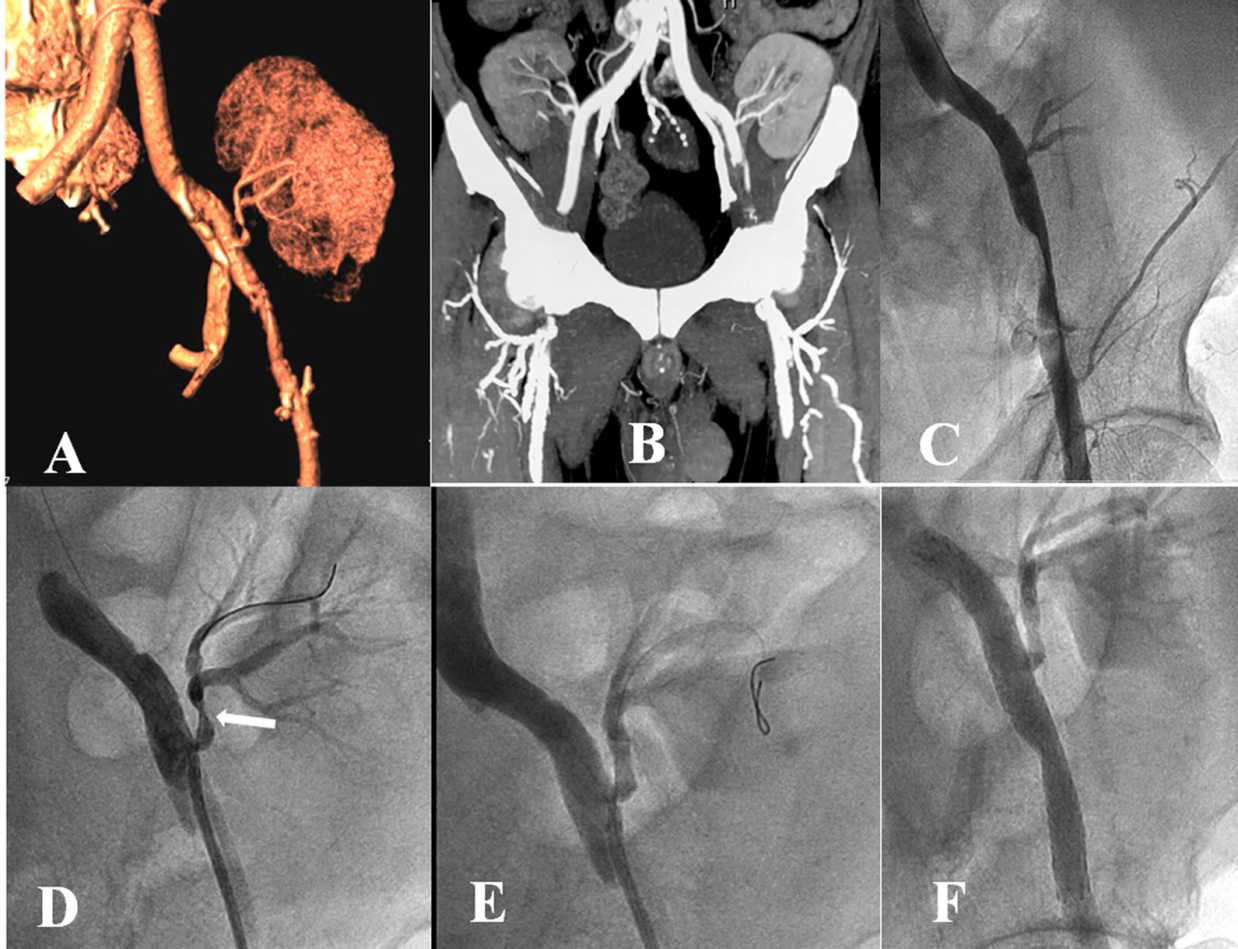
Reconstructed volume-rendered (A) computed tomography angiogram with maximum intensity image projection (B) showed 90% stenosis of the transplanted renal artery (A), and left external iliac artery (EIA) dissection (A) with 90% stenosis (B). Bilateral renal grafts anastomosed to the EIA can be seen (B). The peripheral angiogram showed 90% stenosis with dissection of the left EIA (C) and 90% stenosis of the proximal part of the transplanted renal artery (D, White arrow). Brisk flow was achieved across the transplanted renal artery (E) and left EIA (F) following endovascular intervention.
